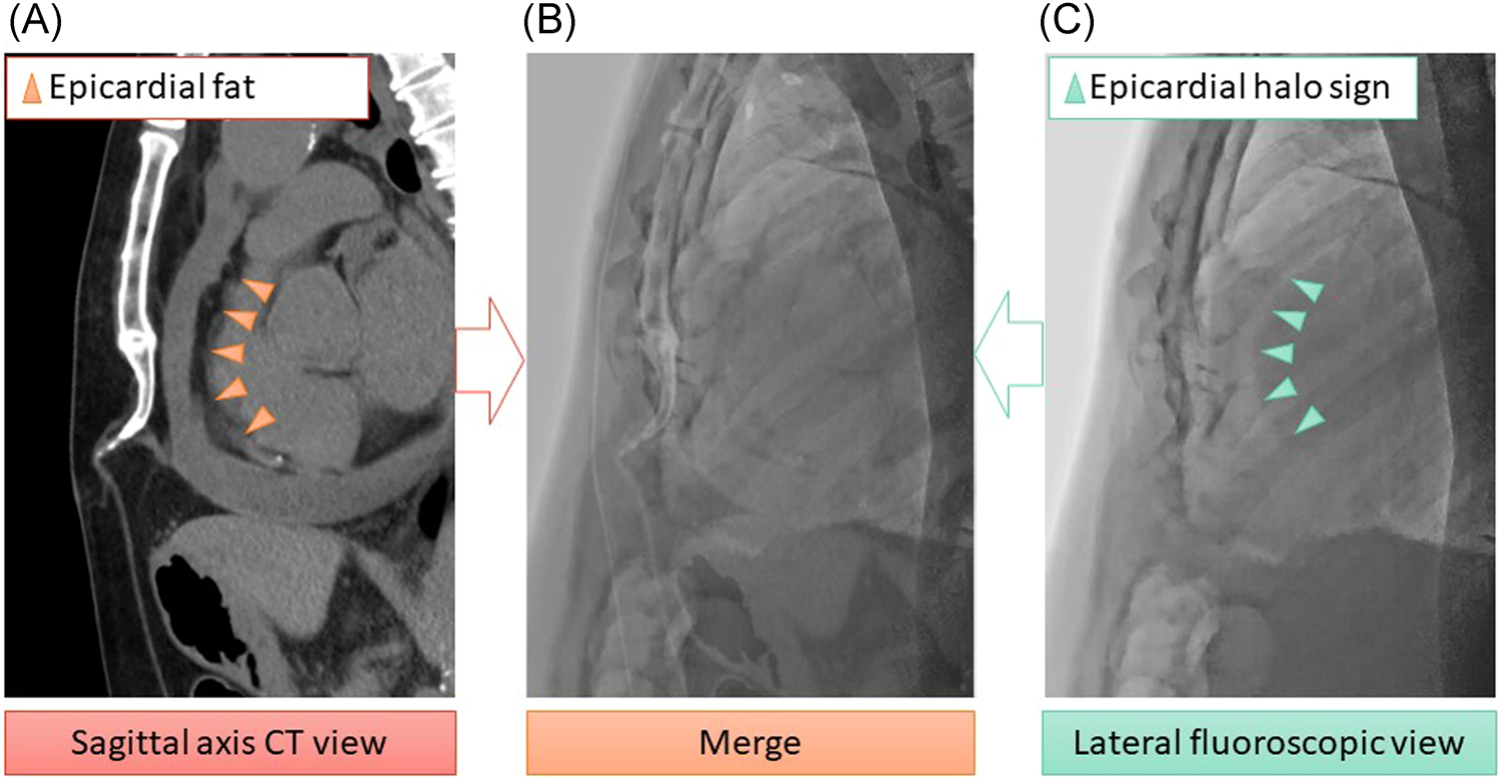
(A) The sagittal axis view along the median line indicates the epicardial fat and a pericardial effusion on the anterior aspect of the heart. (B) A merge view between the sagittal axis CT and lateral fluoroscopic view. (C) The lateral R−L fluoroscopic view exhibits an “epicardial halo sign” in an identical location as the epicardial fat. The anterior aspect of the area with the epicardial halo sign and below the sternum represent the pericardial effusion space. CT, computed tomography
